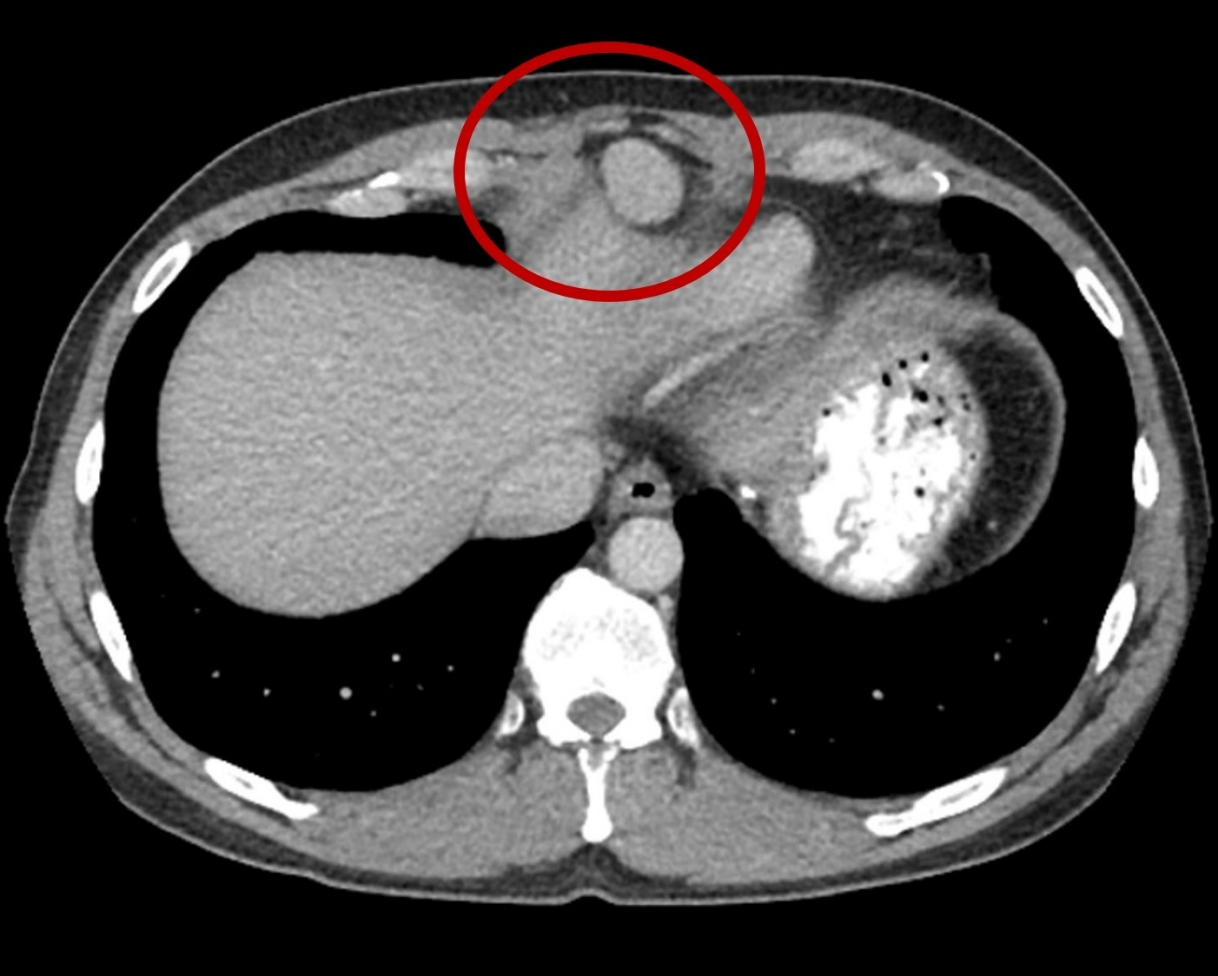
Computed tomography (CT) abdomen showing a solitary circumscribed mass (red circle) in segment 2 of the liver, measuring 2.5 x 2.0 cm and arising exophytically and anteriorly.
